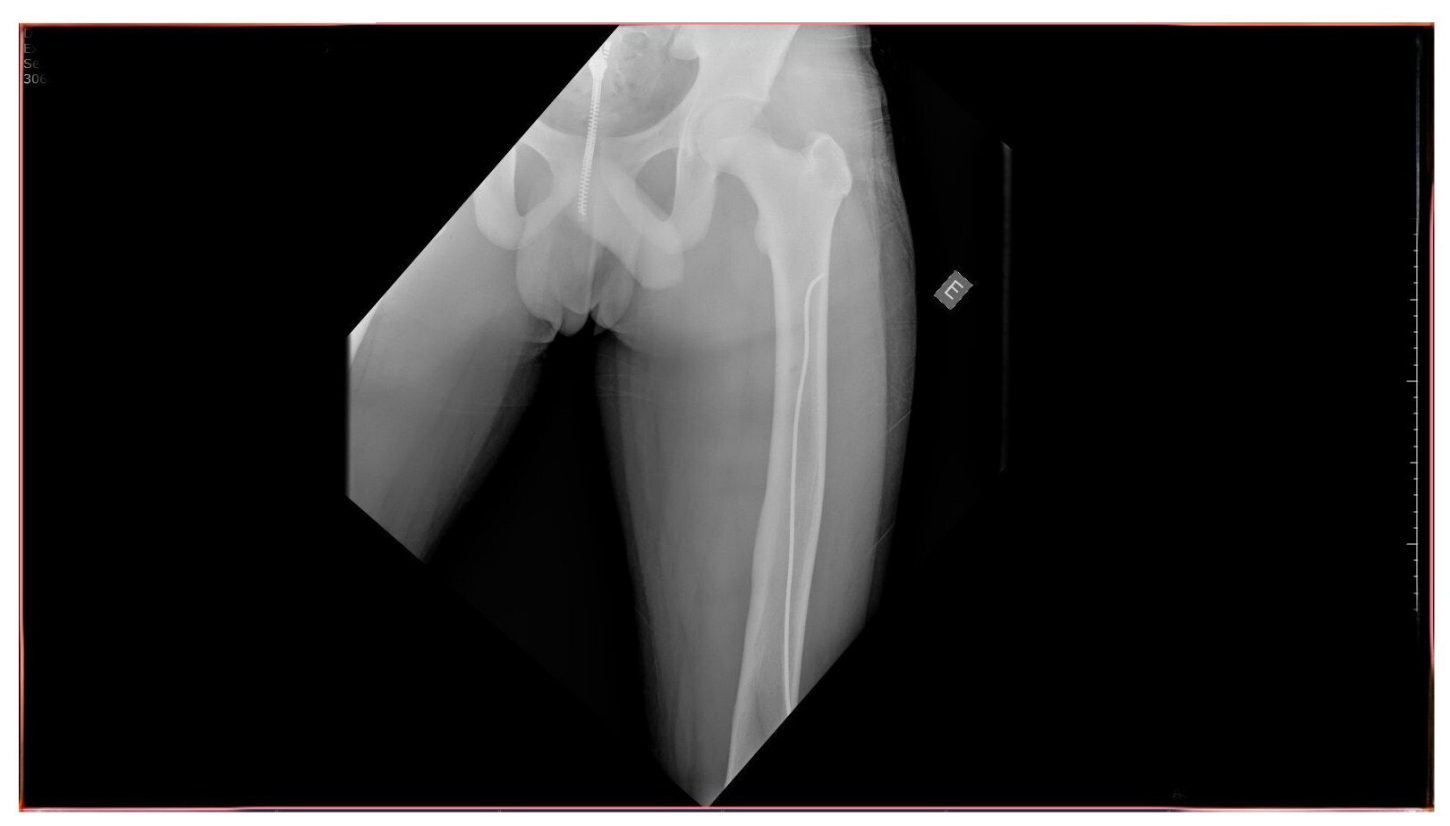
Control radiography conducted 8 months after the procedure showing good bone consolidation and the flexible nail in a normal position within the left femur (1/3) Source: Archives of the Vascular Surgery Department, Hospital Instituto Dr. José Frota.
